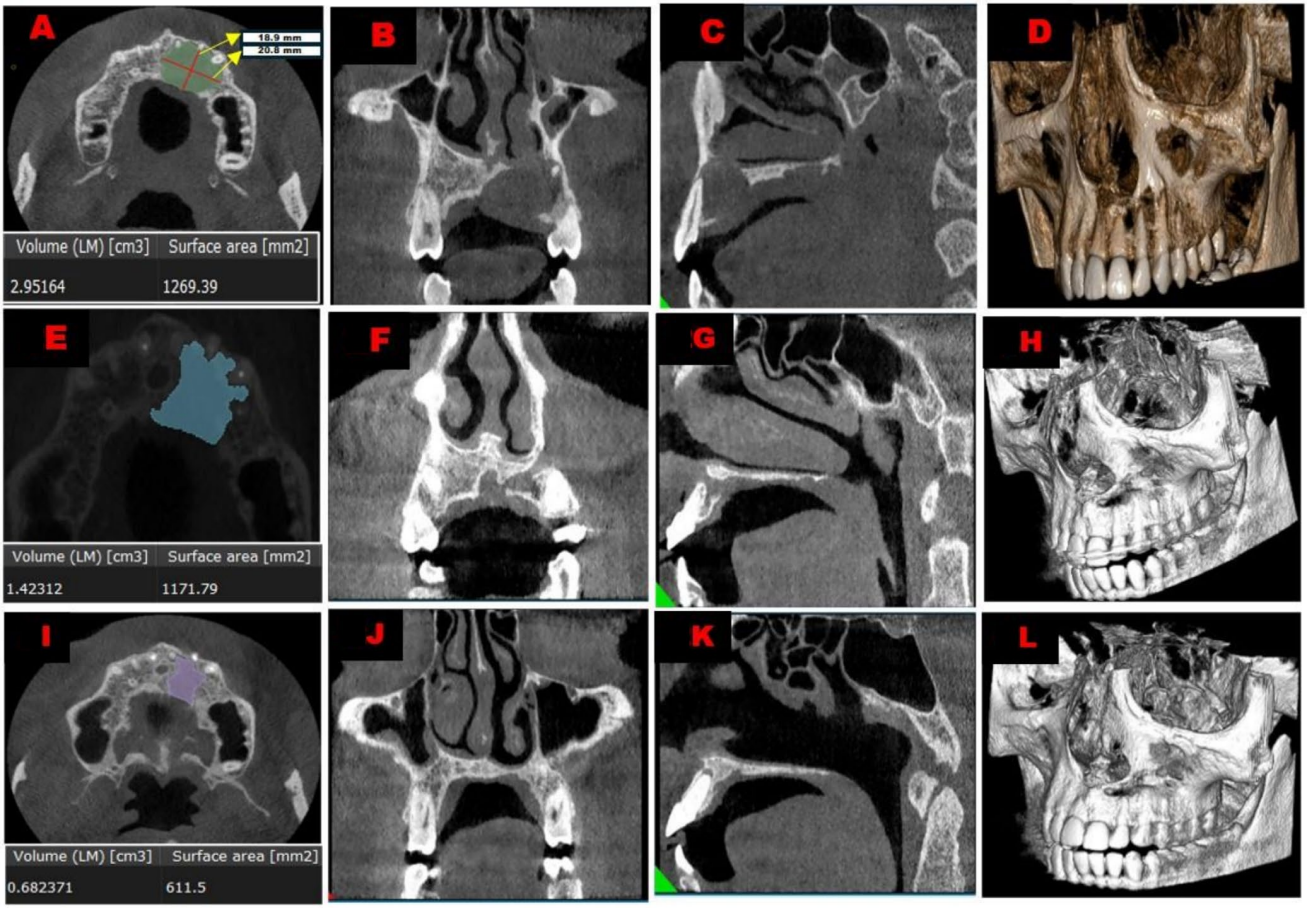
Cone beam computed tomography (CBCT) images. (A) Pre‐operative axial view of the volumetric and surface area analysis along with the maximum diameter of the cystic lesion. (B) Pre‐Operative coronal view of lesion infiltrating the floor of the nasal cavity. (C) Pre‐Operative sagittal view of lesion infiltrating the floor of the nasal cavity. (D) Pre‐operative 3‐Dimensional model showing loss of buccal cortical plate and nasal floor. (E) Axial View of volumetric and surface area analysis of the cystic lesion at 6 months follow up. (F) Coronal view of the lesion at 6 months follow up showing signs of bone formation. (G) Sagittal view of the lesion at 6 months follow up showing signs of bone formation. (H) 3‐Dimensional model of the lesion at 6 months follow up showing signs of bone formation. (I) Axial View of volumetric and surface area analysis of the cystic lesion at 2.5 years follow up. (J) Coronal view of the lesion at 2.5 years follow up showing further signs of bone formation. (K) Sagittal view of the lesion at 2.5 years follow up showing further signs of bone formation. (L) 3‐Dimensional model of the lesion at 2.5 years follow up showing further signs of bone formation.
